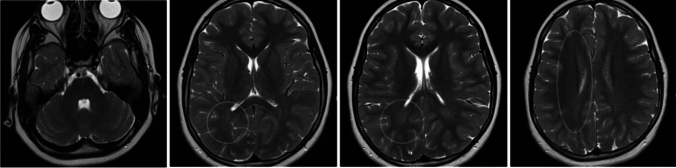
Patient 2’s brain MRI at the age of 5. Notice the posterior T2 white matter hyperintensities evocative of myelination delay (white circles)
